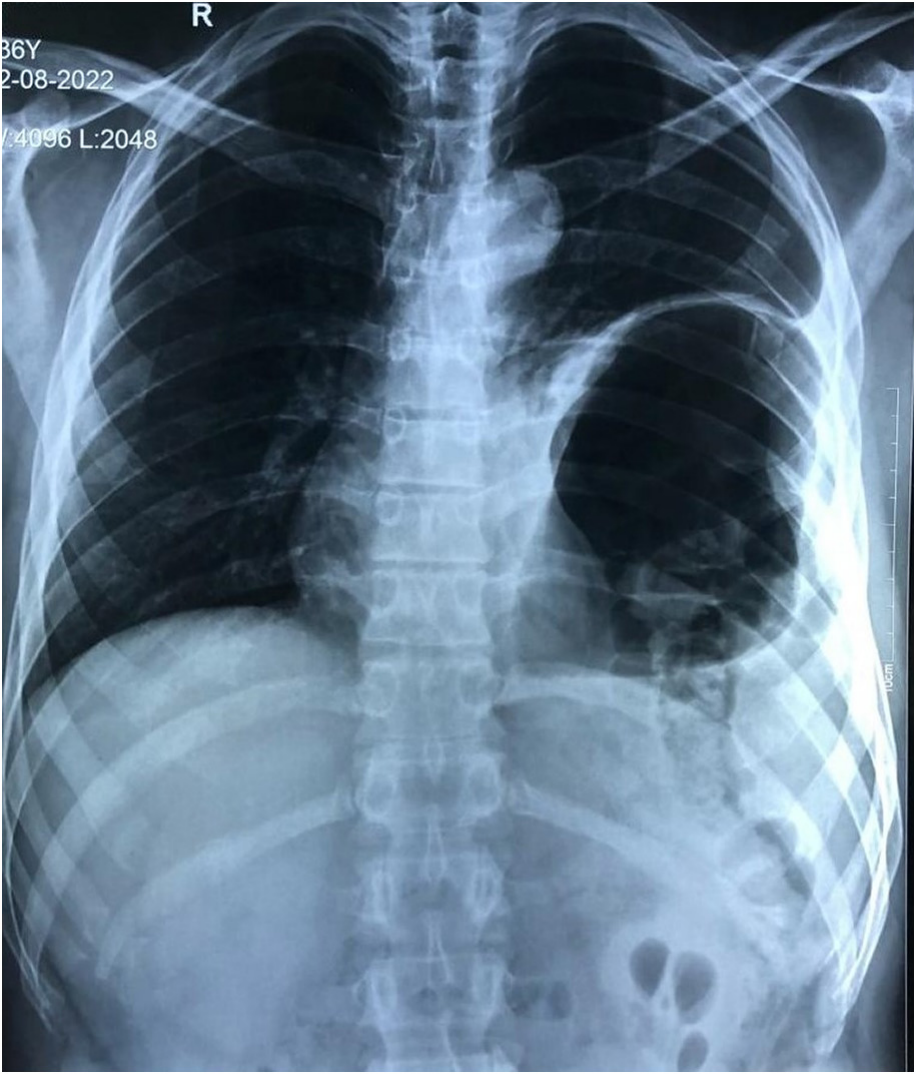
Chest radiograph showing suspected elevation of left hemidiaphragm and air‐filled viscus over the left lower hemithorax.
